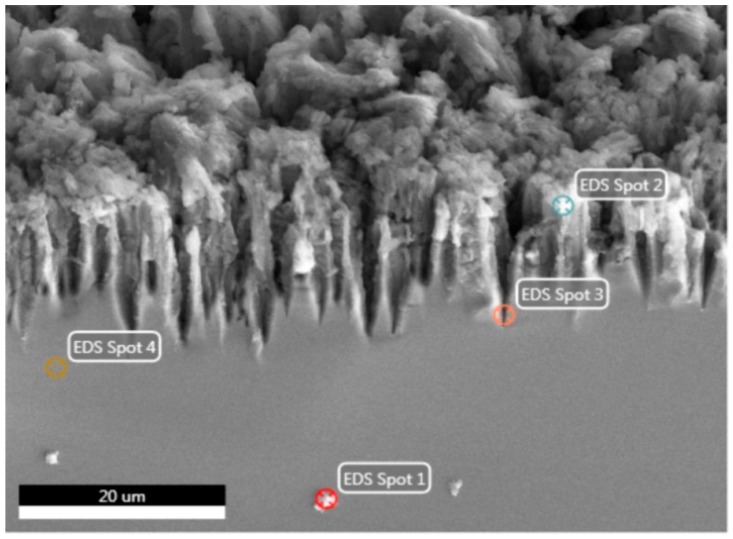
SEM image of porous silicon after electrooxidation and energy-dispersive X-ray (EDX) analysis spots.
